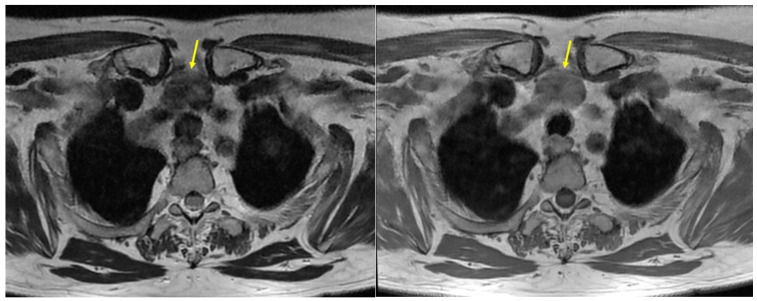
ETTM aspects (yellow arrow): MRI with IV contrast showing a nodule of 2.1 by 2.6 by 3 cm in the pre-vascular compartment of the upper mediastinum on the median line (pre-tracheal): (left) T2 moderate hypo-intensity and (right) T1 iso-intensity.
